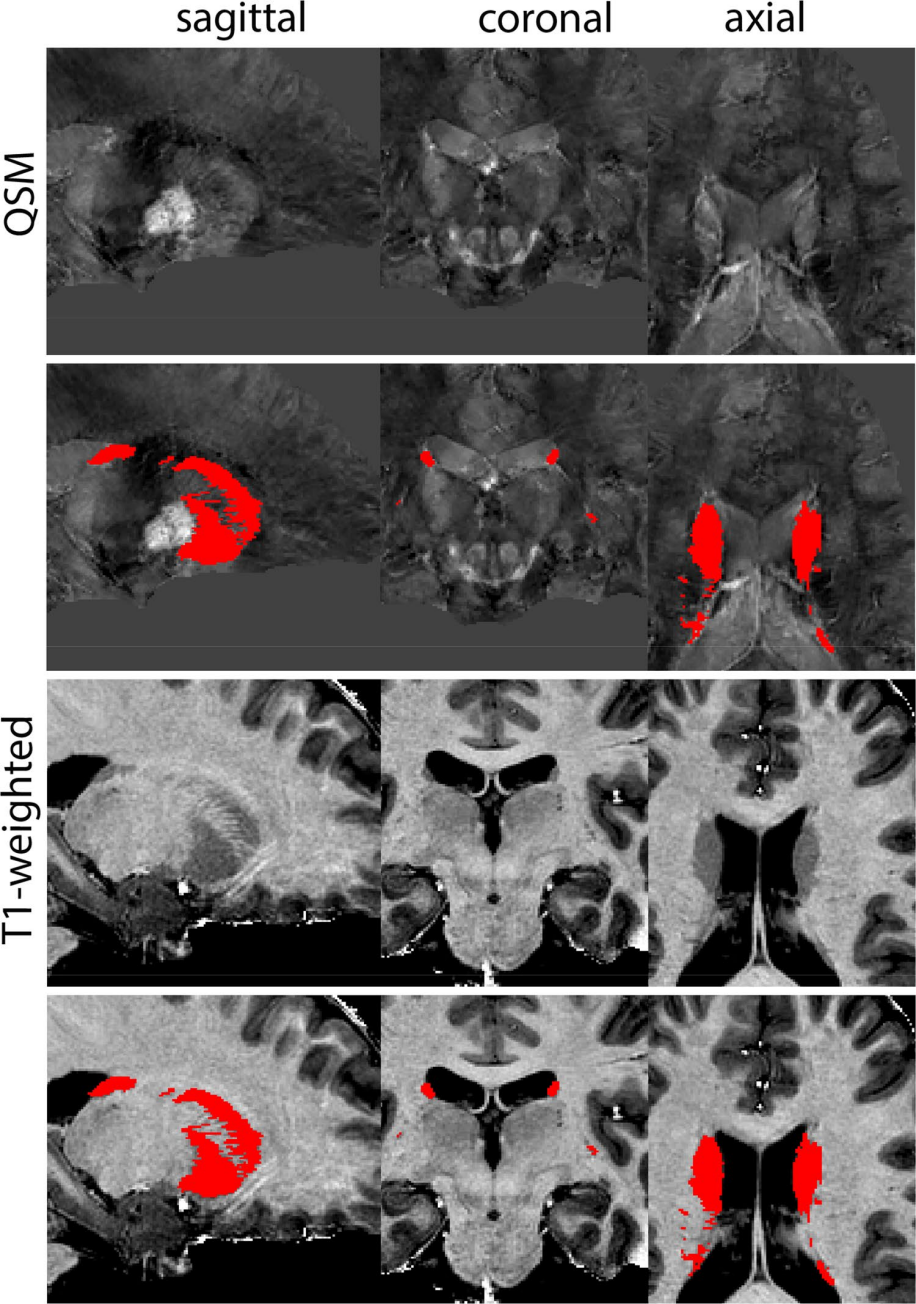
a The striatum (Str) at rostral levels shows the caudate nucleus. Note the clear visibility on T1-weighted contrasts. b The striatum (Str) at somewhat more caudal levels shows the caudate nucleus and the putamen. Note the clear striatal islands in the ic. c The striatum (Str) at even more caudal levels shows STR shifting in lateral direction at the level of the (hypo-) thalamus. d The striatum (Str) becomes patchy and disappears in the coronal plane
